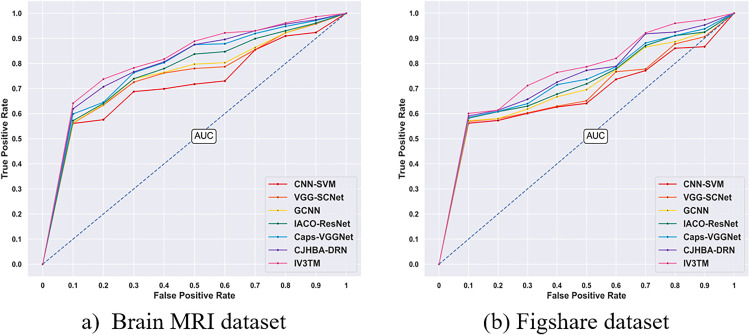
ROC analysis. a) Brain MRI dataset. (b) Figshare dataset.
